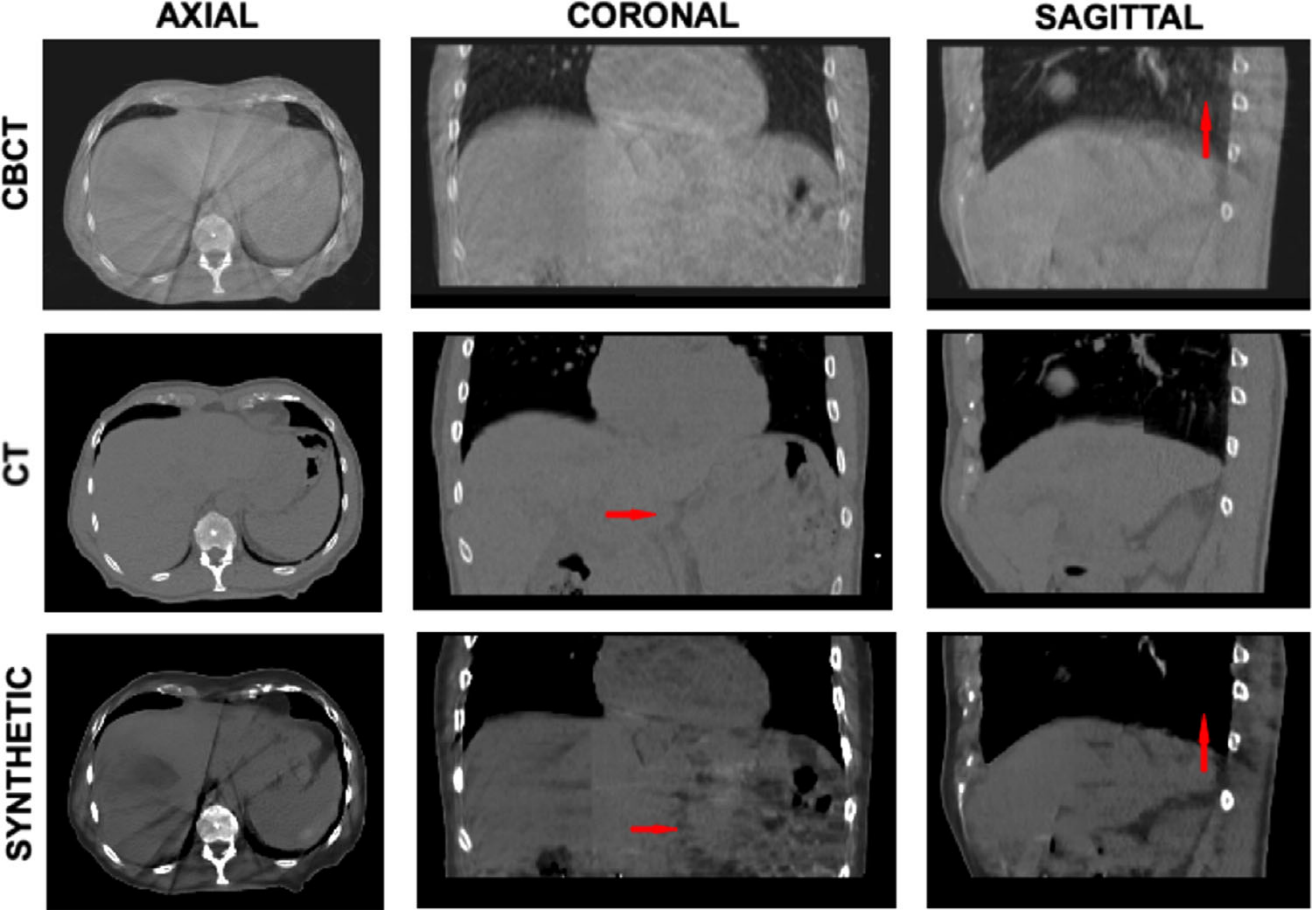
Image quality visualization. Cone beam computed tomography (CBCT) (top W:541 L:116), computed tomography (CT) (middle W:541 L:116), and synthetic CT (bottom W:541 L:116) images from the patient of interest (test patient). Axial, coronal, and sagittal slices from left to right in that order. Red arrows on the sagittal and coronal slices pointing at lung artifact reduction and improved resolution, respectively.
